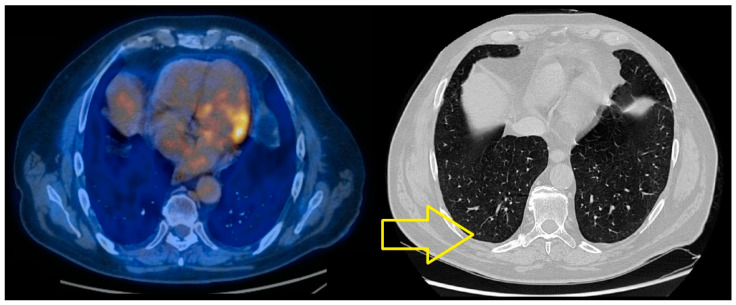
PET-CT scan—June 2022. Dimensional regression of the bilateral micronodular and nodular lesions, with a current maximum of 3 mm (yellow arrow).
